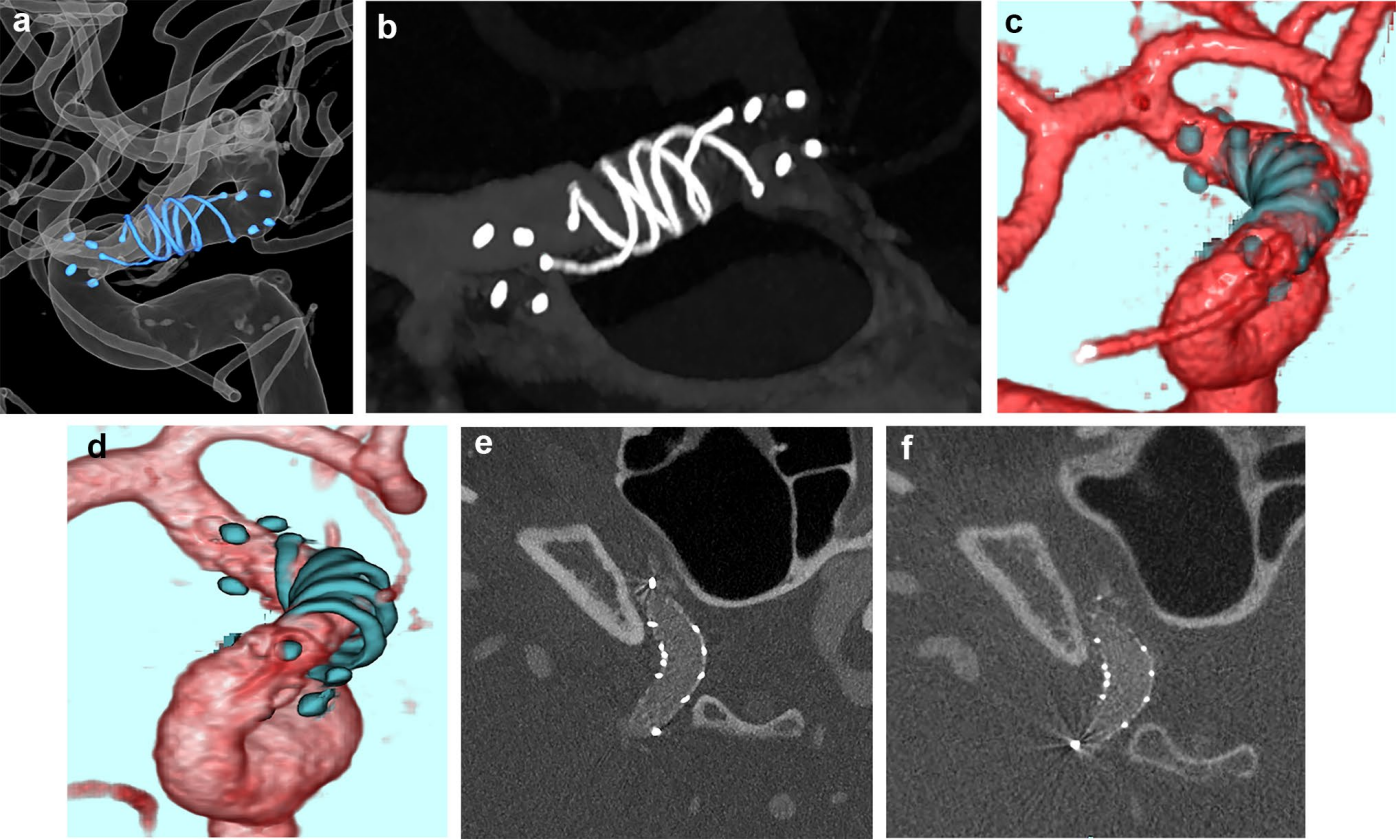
Cerebral aneurysm treated with a flow diverter stent. This case involved a woman in her 40 s who presented with a headache. The MRI revealed a 2.5-mm aneurysm with a broad neck (6 mm) on the anterior wall of the right internal carotid artery at the C2 segment. To treat the aneurysm, a flow diverter stent (FRED 4/18 mm) was implanted to treat the aneurysm (a). Both EID-CTA and PCD-CTA (kernel Hv89) were performed after flow diverter stenting. The PCD-CTA clearly showed the stent mesh, with image resolution comparable to that of traditional angiography (b). When comparing the 3D images from EID-CTA (c) and PCD-CTA (d), PCD-CTA was superior for evaluating the stent’s shape. The PCD-CTA also showed evidence of good apposition between the stent and vessel wall, with no thrombus formation in the stent (e: axial image). The resolution achieved with PCD-CTA was equal to that of angiography with cone-beam CT and diluted contrast media (f: axial image). *3D* three-dimensional, *CT* computed tomography, *MRI* magnetic resonance imaging, *EID-CTA* energy-integrating detector-computed tomography angiography, *PCD-CTA* photon-counting detector-computed tomography angiography
